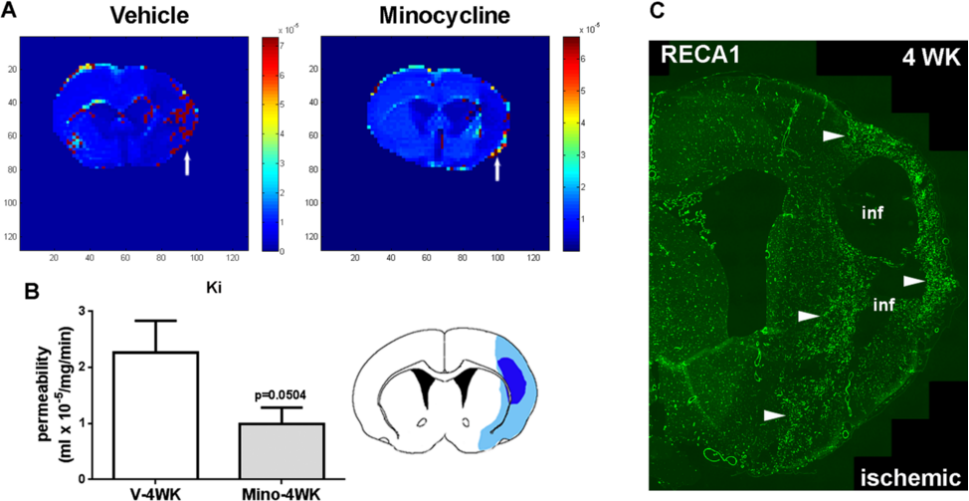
Blood–brain barrier permeability monitored by magnetic resonance imaging. A. Parametric image *K*
